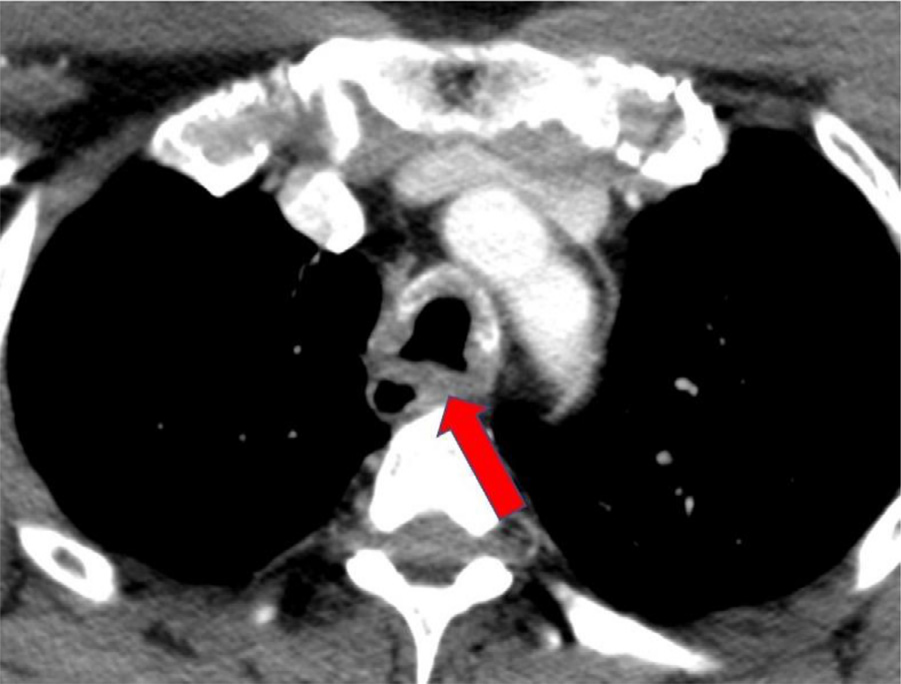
Axial contrast-enhanced CT of the chest at a level above the carina demonstrates circumferential thickening and calcification of the distal trachea without sparing of the posterior wall.
